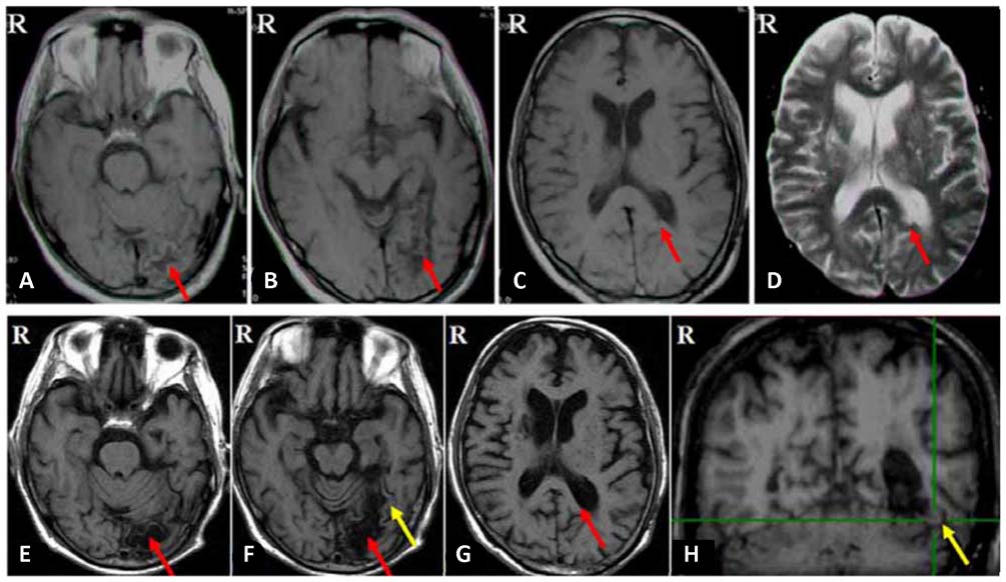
Lesions and intact VWFA shown in the structural MRI of the patient's brain. The red arrows indicate the infarctions in the left ventral medial occipitotemporal cortex (A, B, E and F) and the left splenium of the corpus callosum extending to the left major forceps (C, D and G). The yellow arrow indicates the intact left lateral mid-fusiform cortex and occipitotemporal sulcus (F and H). The VWFA is highlighted with the green crosshair (Talairach coordinates: x = −43, y = −54, z = −12) in a coronal slice of spatially normalized SPGR images (H).
